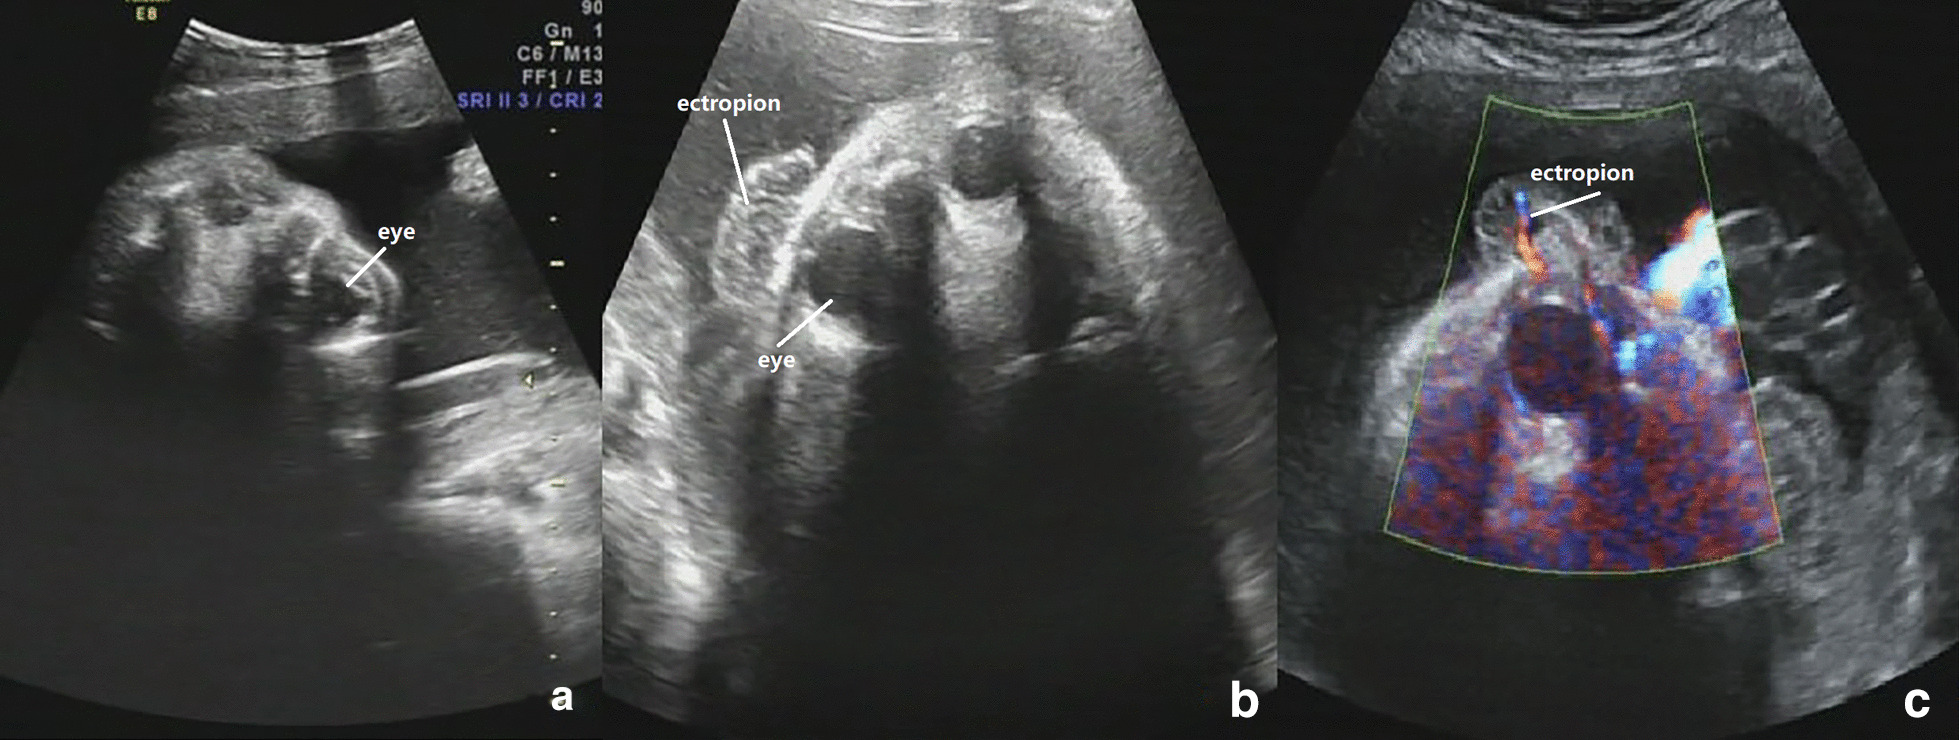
a 2-D ultrasound: no abnormality was observed in both eyes of the fetus at 27 weeks. b 2-D ultrasound: fetal ectropion at 31 weeks. c Color doppler display: blood flow signals from the eyes are detected in the everted eyelid
